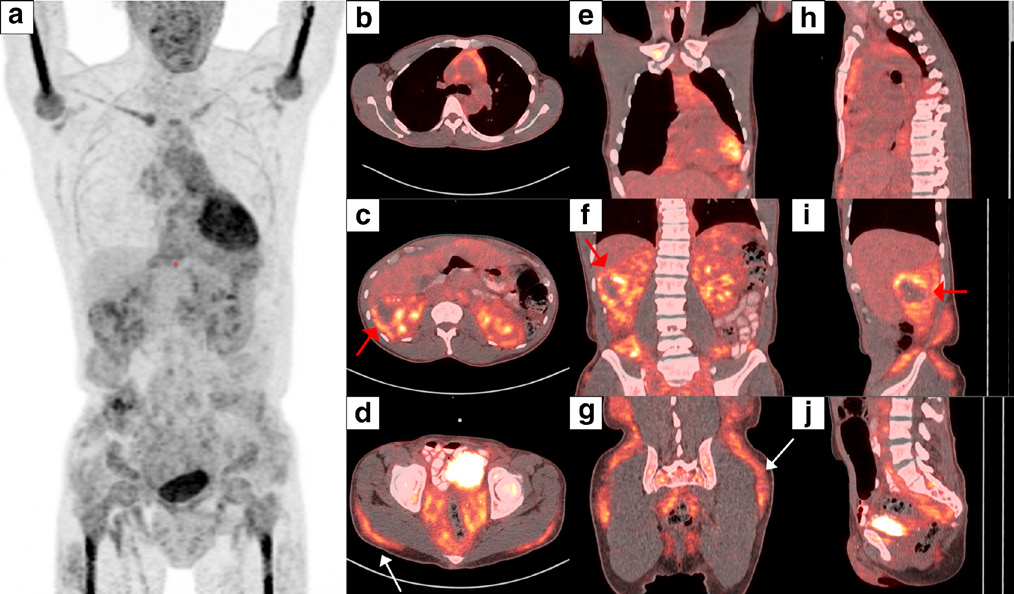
F-18 FDG PET CT. Maximum intensity projection image (a) demonstrating low-level metabolic activity (SUV_max_ up to 5) associated with diffuse infiltrative soft-tissue density in the mediastinum, abdomen and pelvis. Fused axial (b), coronal (e) and sagittal (h) images of the chest demonstrate low-level FDG uptake in the anterior mediastinum. Fused axial (c) coronal (f) and sagittal (i) images of the abdomen show low-level FDG avidity in the infiltrative soft-tissue of the retroperitoneum. Heterogeneous peripheral increased FDG uptake in the right renal mass (Red arrow). Fused axial (d), coronal (g) and sagittal (j) images of pelvis show FDG uptake in soft-tissue infiltrate in the mesorectal fat and deep subcutaneous fat overlying the gluteal muscles (White arrows).
